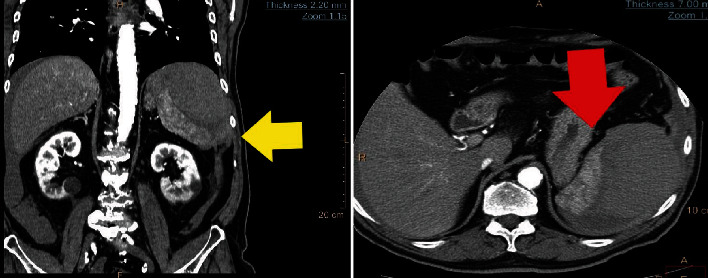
(a) Coronal CT depicting the ruptured spleen (yellow arrow) with concomitant bleeding in the left upper quadrant. (b) The ruptured spleen (red arrow) in axial CT.
